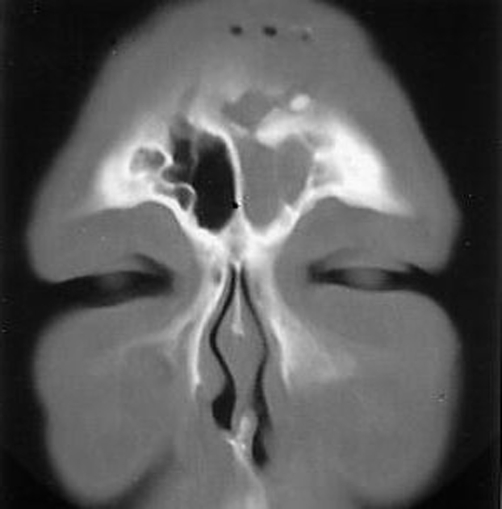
CT scan of the para nasal sinuses showing a left ethmoid sinus foreign body and opacification of all sinuses on the left.
